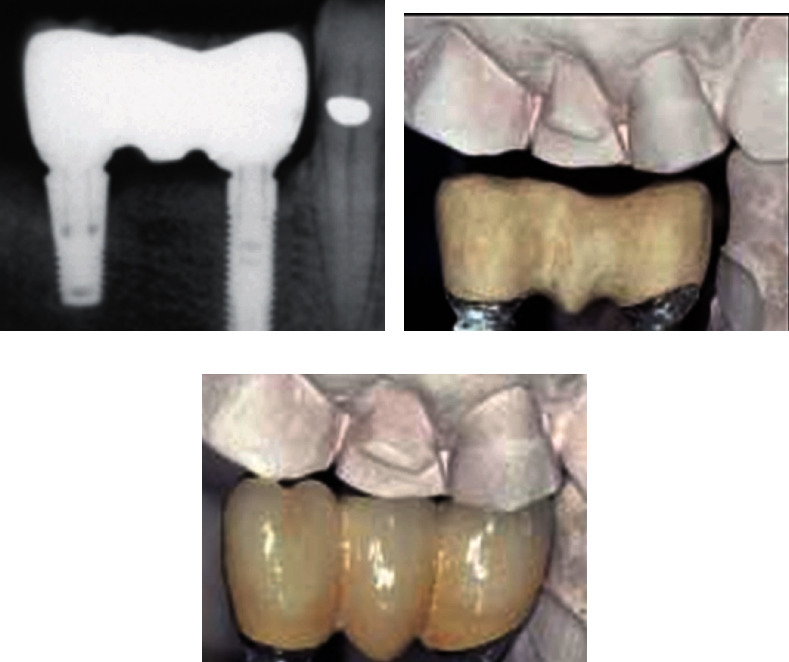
(a) Radiographic image, (b) frame, and (c) In-Ceram Zirconia (InZ) [52].
